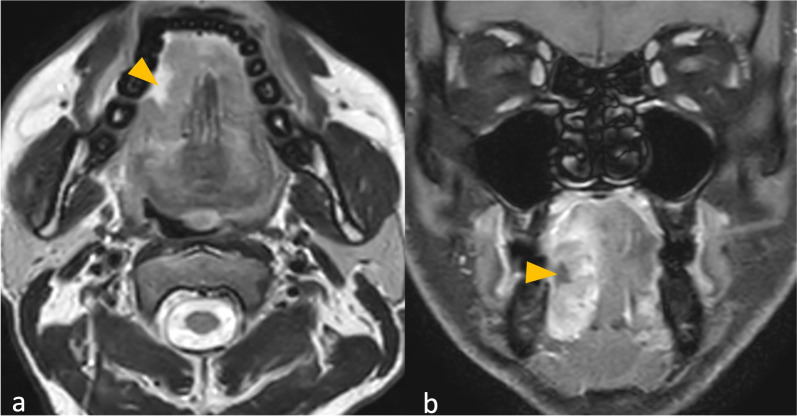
(a, b) MRI images of oral tongue squamous cell carcinoma with tumor ulceration. Axial T2 Weighted Imaging (T2WI) (a) and coronal contrast-enhanced (CE) fast spoiled gradient echo (FSPGR) image (b) show breach of mucosa overlying the tumor, suggestive of tumor ulceration (arrowheads).
